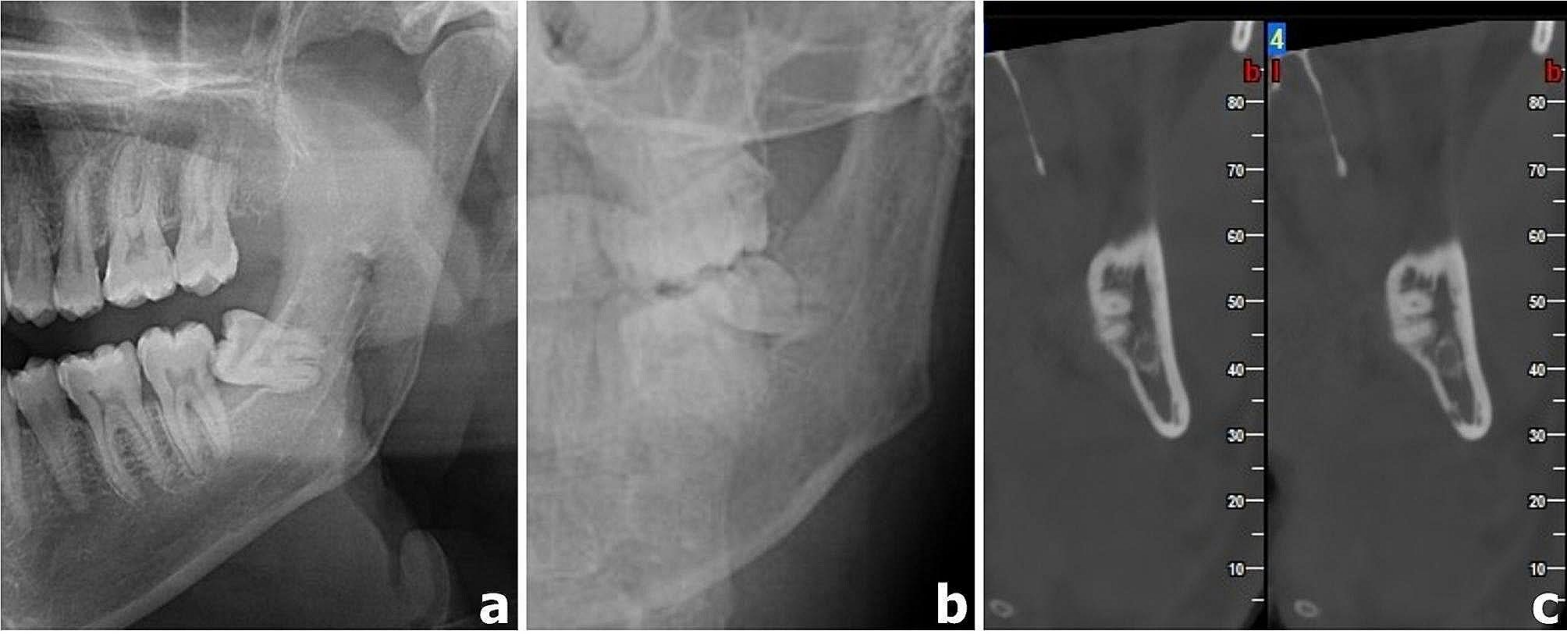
Cropped PR showing the roots of MM3 in relation to the IAN (a), cropped PA radiograph (b), and cross sectional CBCT images (c) of the same patient showing the absence of a relationship between MM3 roots and IAN
